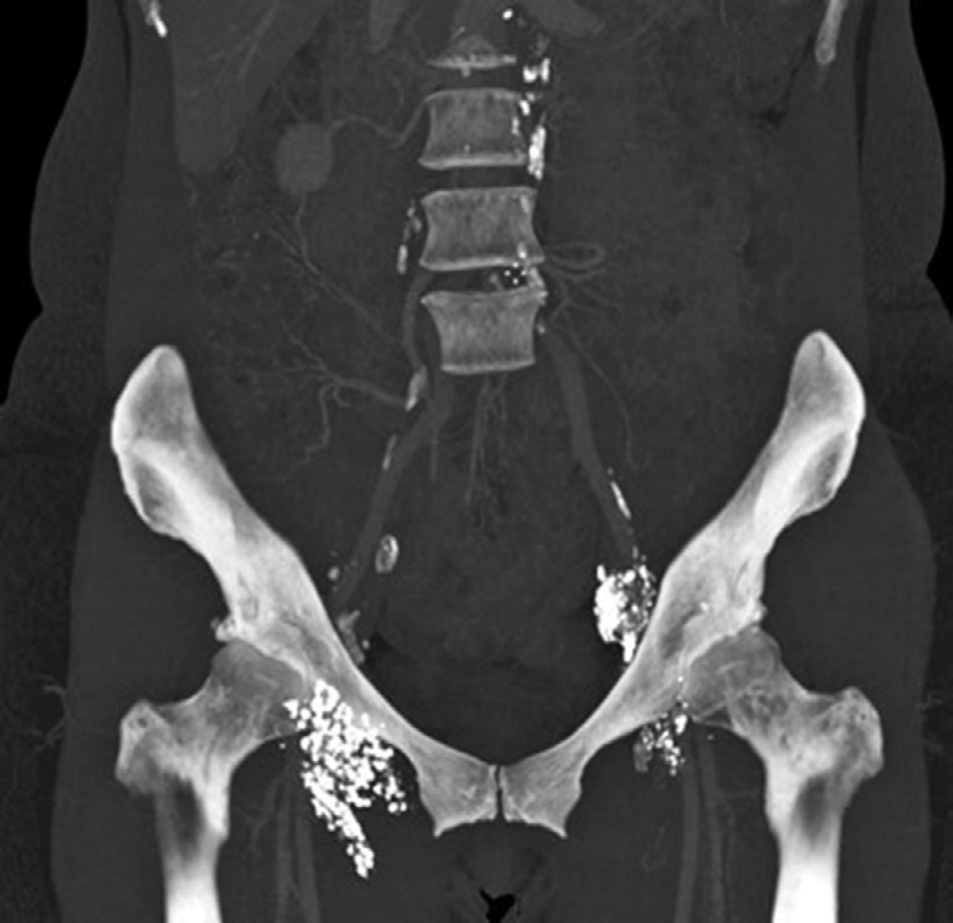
Coronal view of control computed tomography image generated approximately six months after transafferent nodal embolization (TNE). The image shows TNE-related radiopaque material next to the inguinal lymph nodes
